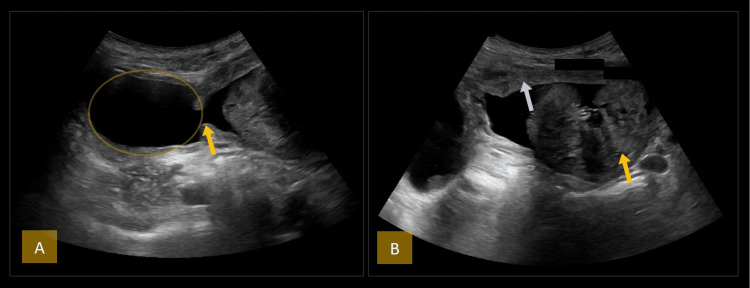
Ultrasonographic evaluation of the bladder and prostate. (A) The yellow arrow indicates the neck of the bladder diverticulum. The yellow circle highlights the right lateral wall bladder diverticulum. (B) The yellow arrow highlights a markedly enlarged median prostatic lobe protruding into the urinary bladder. The white arrow points to diffuse bladder wall thickening consistent with bladder outlet obstruction.
